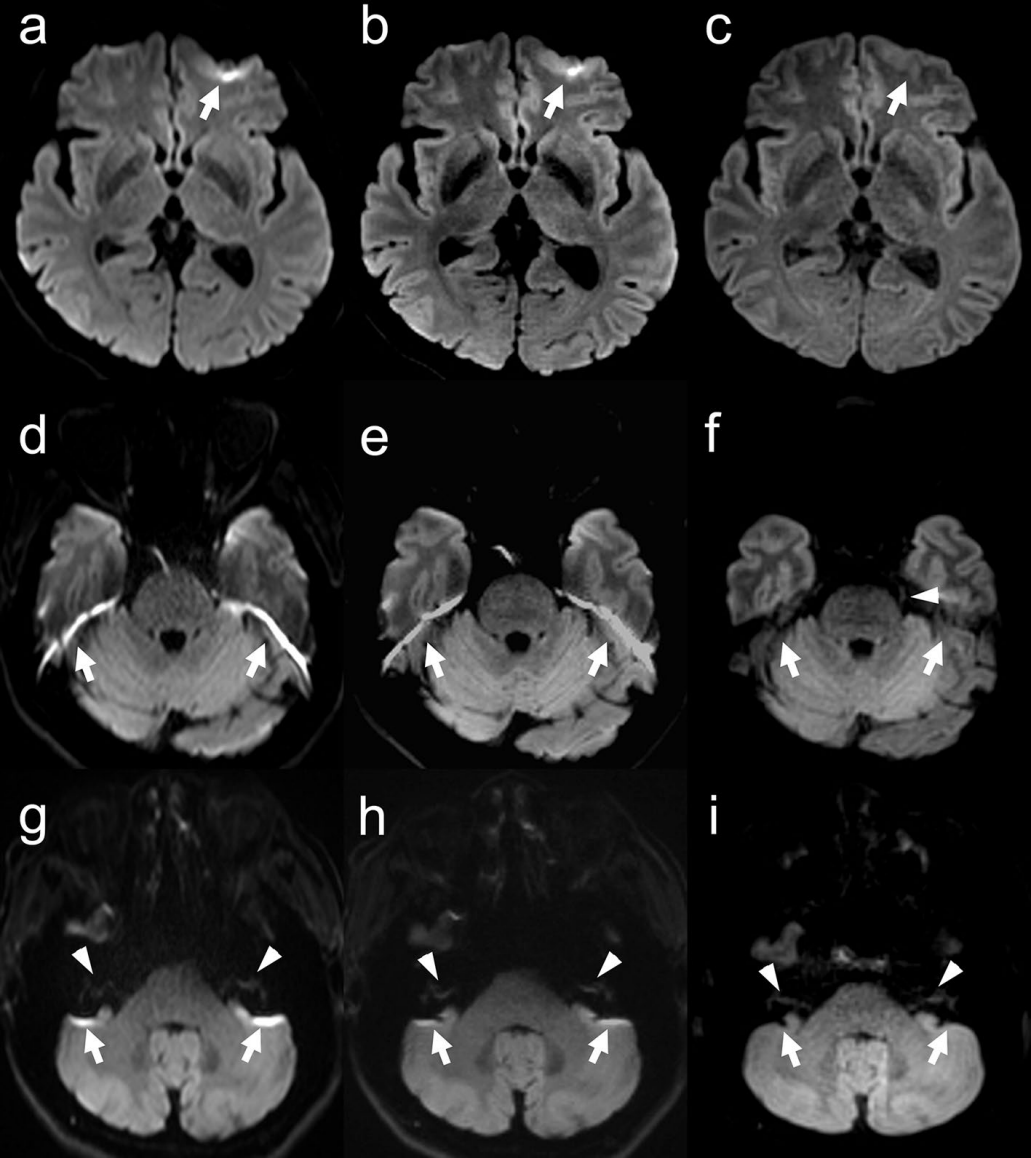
Representative images of SS-EPI DWI (a, d, g), RESOLVE DWI (b, e, h), and TGSE-BLADE DWI (c, f, i) in a 67-year-old healthy female volunteer. The arrows indicate susceptibility artifacts. Artifacts are seen near air–bone interfaces in RESOLVE DWI and SS-EPI DWI, whereas few artifacts are seen in TGSE-BLADE DWI. The trigeminal nerve (arrowhead) in visualized in TGSE-BLADE DWI (f) but is blurred in RESOLVE DWI and SS-EPI DWI. The vestibulocochlear nerve (arrowheads in g–i) are best visualized in TGSE-BLADE DWI.
